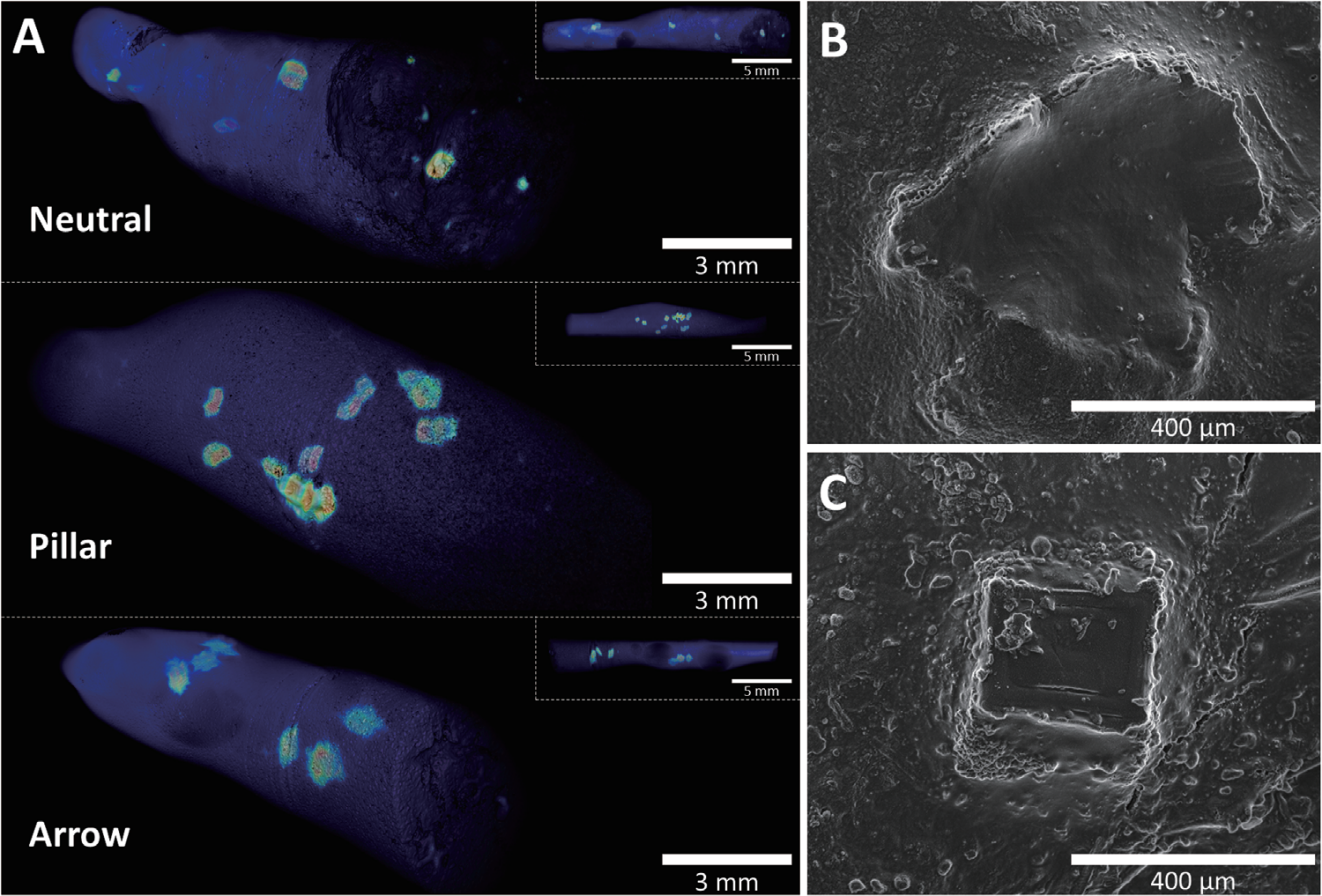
Spatial dynamics and mucosal interactions of microcontainers. A) CT scan images of neutral, pillar, and arrow microcontainers inside small intestinal pieces, revealing their spatial dynamics. Accompanying CT scans movies are found online (Movies S1–S3, Supporting Information). B,C) CryoSEM images of pillar microcontainers showing their mucosal interactions, such as embedment into the intestinal tissue and the microcontainer orientation.
